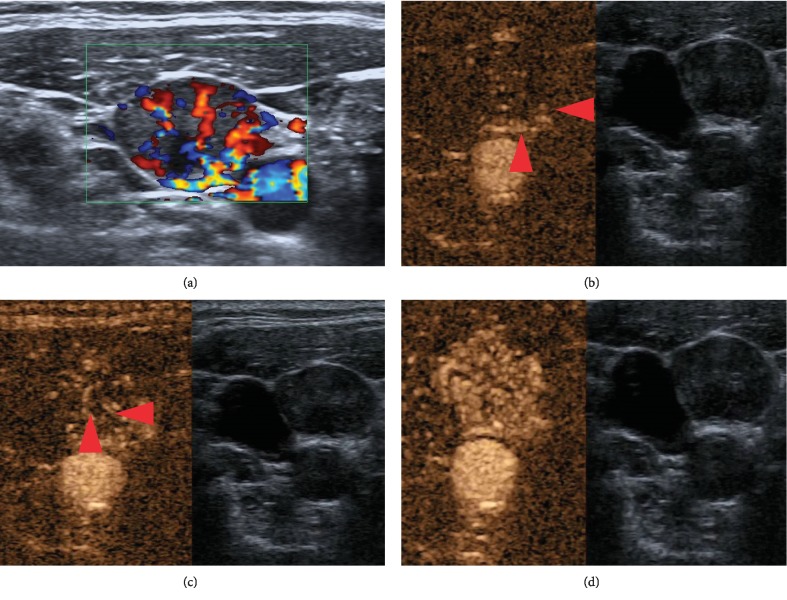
Fifty-one-year-old PTC woman had received left thyroidectomy two years ago. ACLNs were detected on the right neck, located in level IV. (a) Loss of fatty hilum, hyperechogenicity, and oval in conventional US (17 × 10 mm). A peripheral (Type IV) vascular pattern was shown in CDFI. Compared with previously conventional sonographic figures, aberrant vessels were clearly shown in chronological order in CEUS figures. (b) Microbubbles rose at the nodal capsule (red arrows) in the beginning (11 s after SonoVue injection). (c) Then aberrant vessels developed from the nodal capsule to hilum (red arrows, 13 s after SonoVue injection). (d) LN showed hyperenhancement 18 s after SonoVue injection. And this ACLN was proved to be metastatic PTC by FNA.
